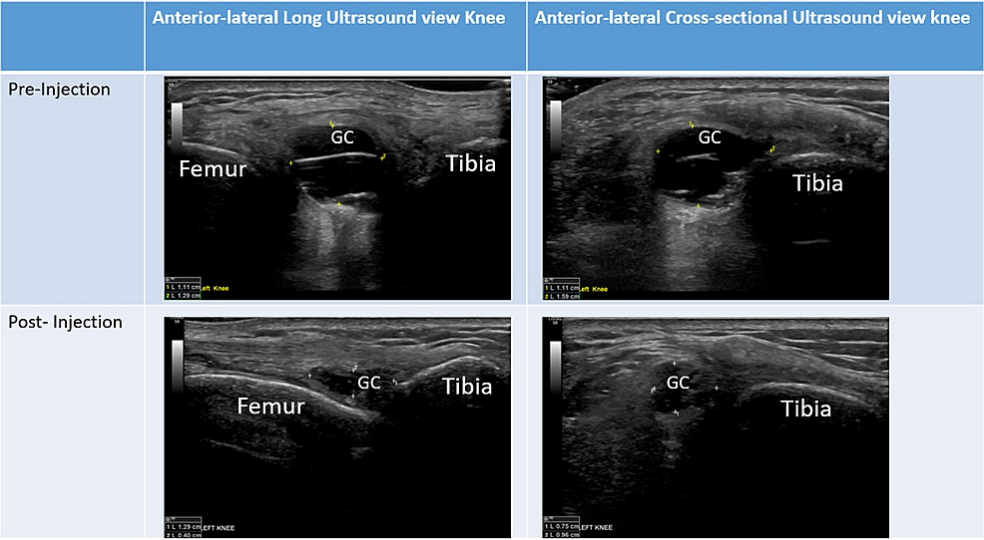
Lateral infrapatellar views acquired with the knee placed at 30° flexion. Left panels: Lateral long axis (sagittal plane) scans with the probe place proximally on the femur and distally on the tibial. Top left preinjection panel: An anechoic ganglion cyst (GC) that has posterior acoustic enhancement over Hoffa’s fat pad as well as septations within the cyst. Top right panel: Orthogonal views in the transverse plane demonstrating the ganglion cyst adjacent to the tibia with similar ultrasound characteristics. Bottom two panels: Postinjection images acquired in the same location and demonstrating reduction in the size of the ganglion cyst GC: ganglion cyst
